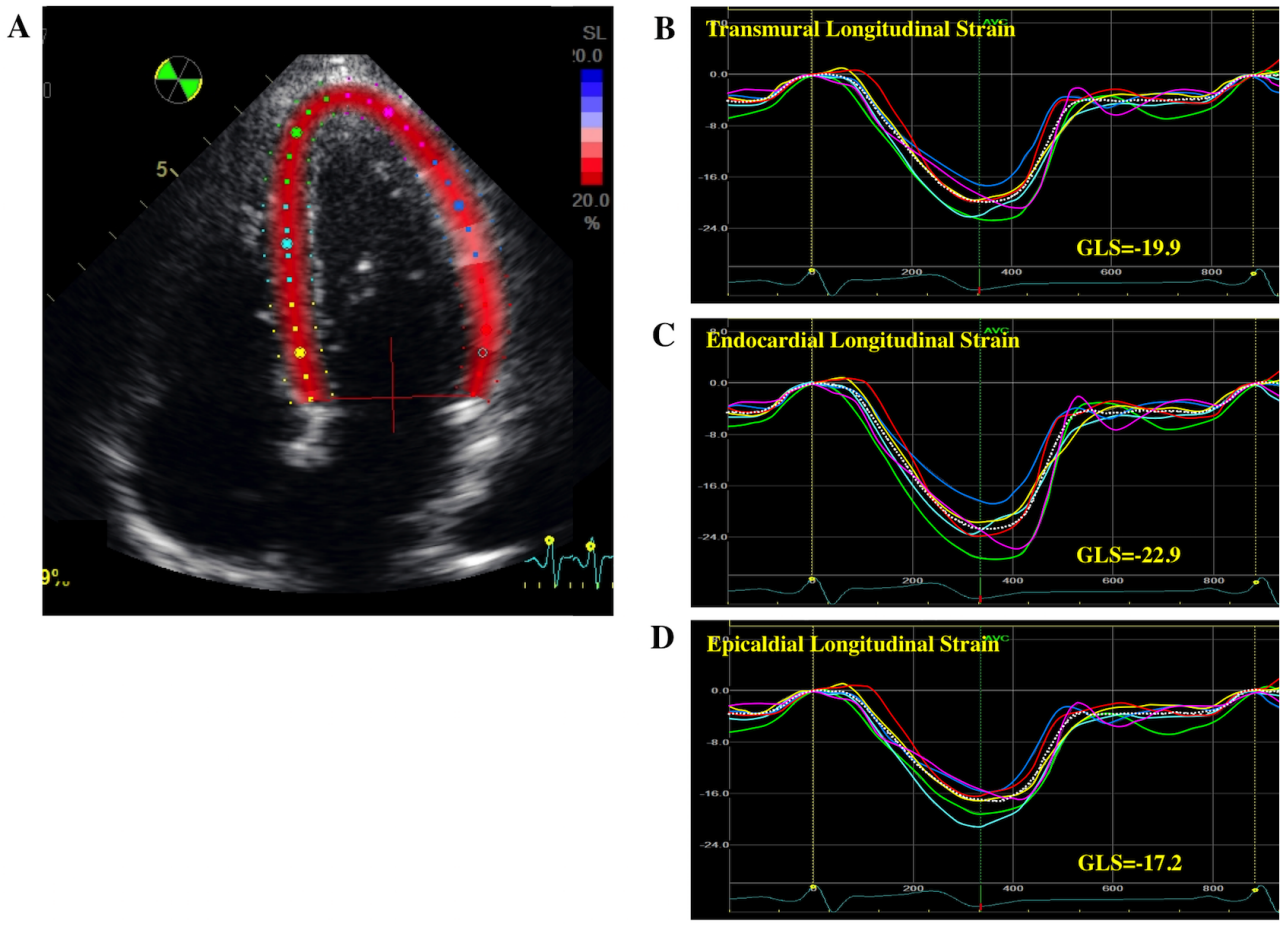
Representative layer-specific speckle tracking analysis of longitudinal strain. A: an apical 4 chamber view with color coded regions of interest, B: transmural longitudinal strain (LS) curve, C: endocardial LS curves, D: epicardial LS curves.
